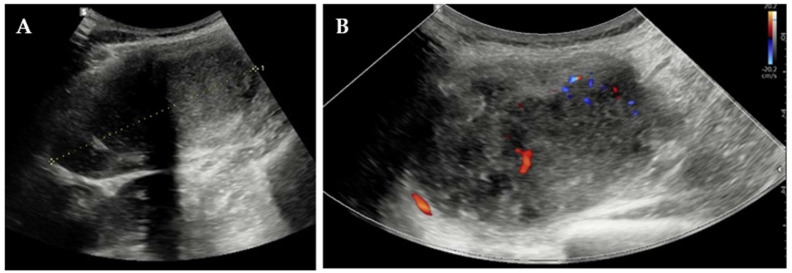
Pre-operative transabdominal US: (A) measurement of the lesion and (B) color-Doppler vascularization.
